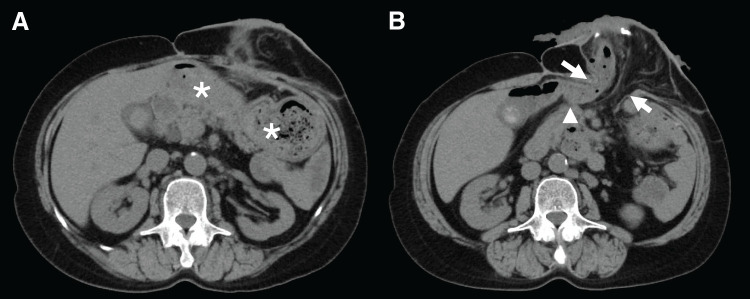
Postoperative CT obtained 2 months after surgery. (A) The stomach (*) is located intraperitoneally beneath the abdominal wall and shows no evidence of protrusion or herniation into the parastomal defect. (B) Only the elevated colon and its mesentery (arrows) are seen protruding through the abdominal wall as the stoma, with no evidence of parastomal hernia recurrence or stoma prolapse. Although the mesh itself is not clearly visualized, the proximal colon (arrowhead) courses along the abdominal wall.
